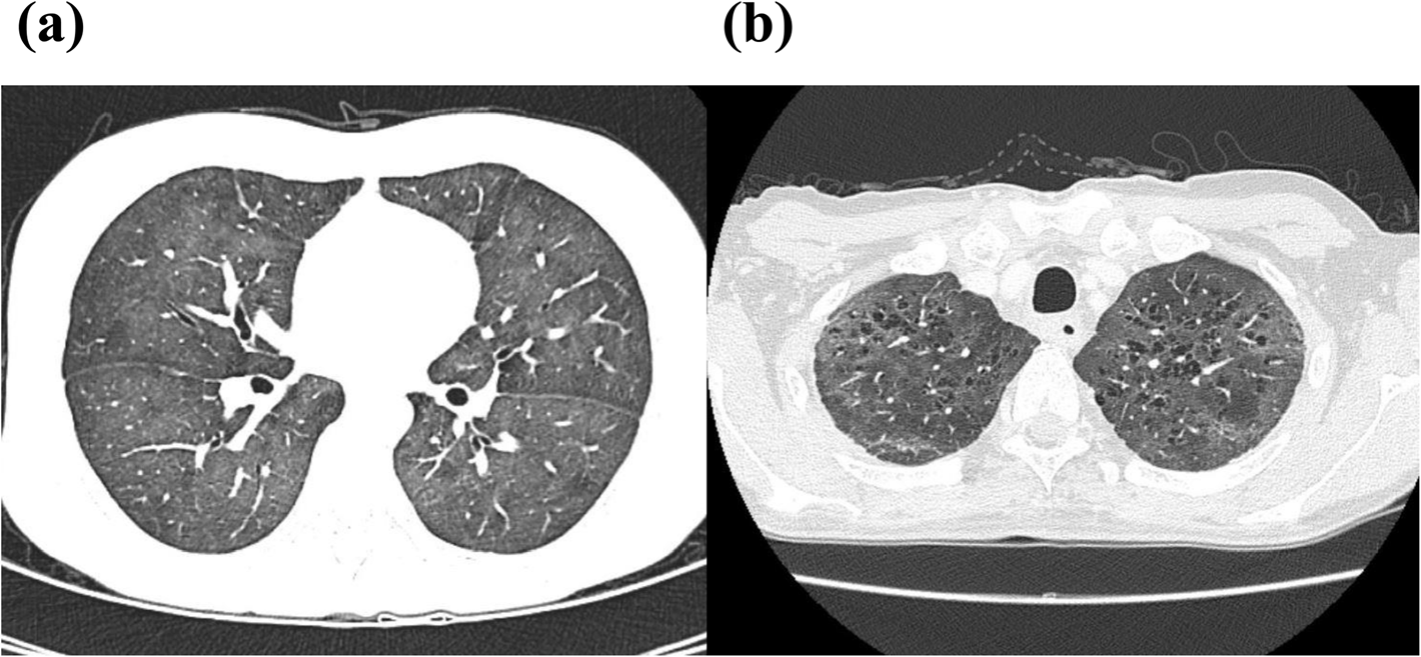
Chest CT findings in patients with ground glass opacities. Patient No. 13 (a) and Patient No. 14 (b) shown in Table 3
